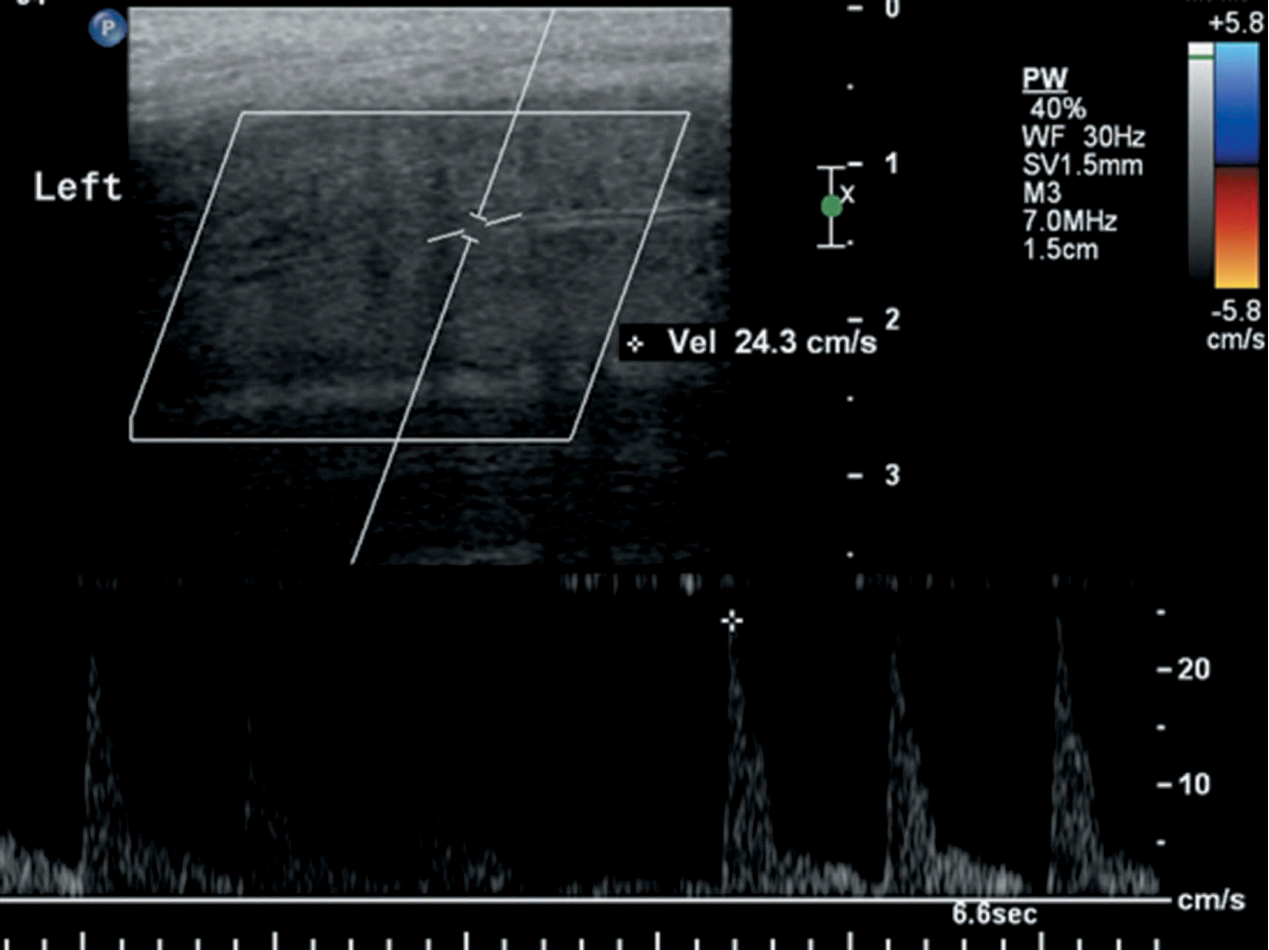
Ultrasound Doppler of the penile artery showing adequate flow after continuous phenylephrine infusion.
